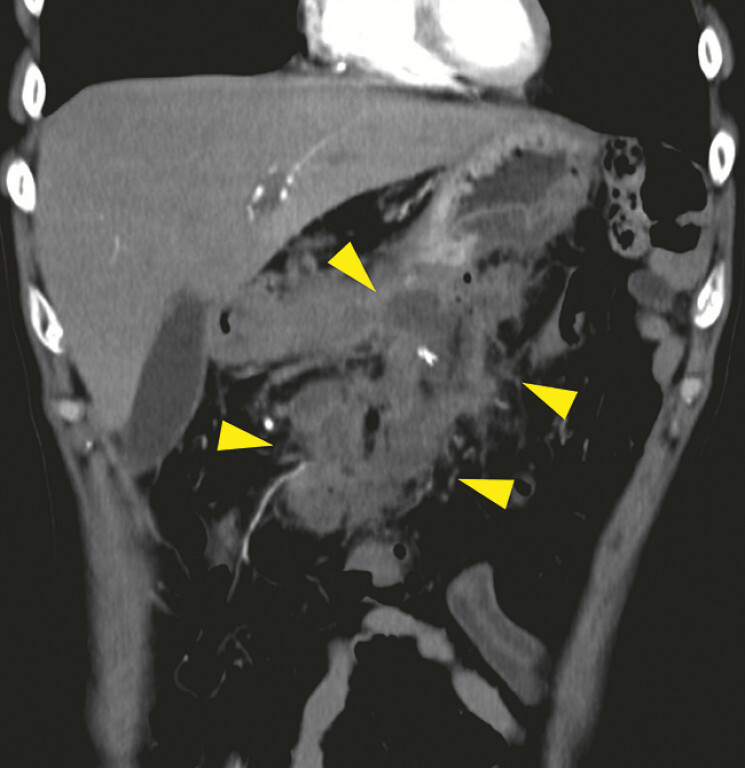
Contrast-enhanced computed tomography showed large post-operative pancreatic fluid collection (yellow arrowheads).
